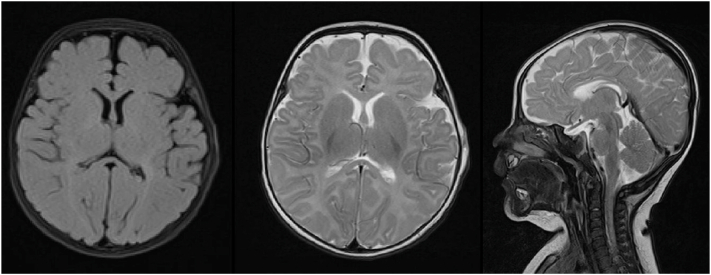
Magnetic resonance imaging (MRI) showed bilateral dilation of the frontotemporal subarachnoid space and bilateral hyperintense lesions of the putamen and thalamus.
